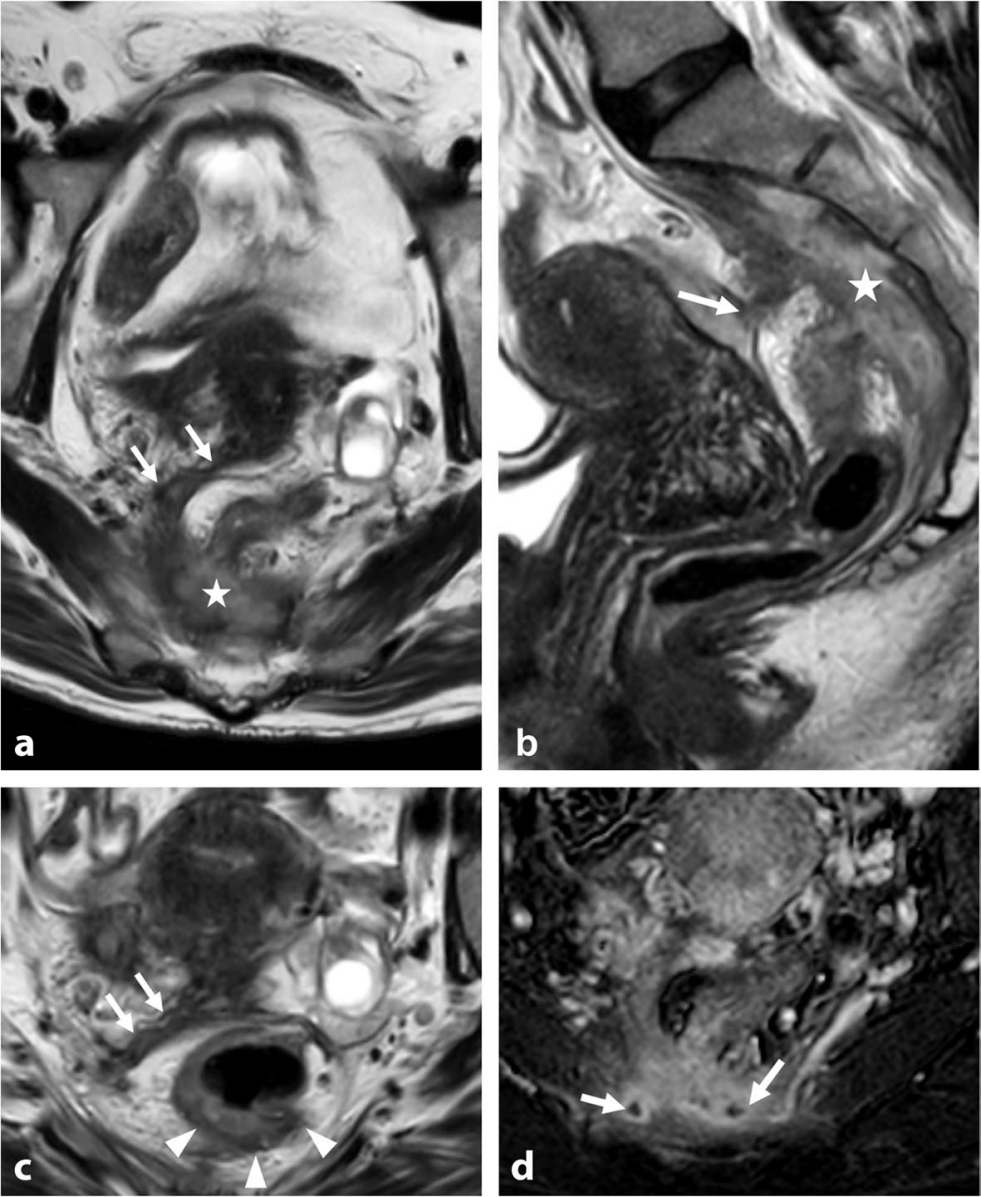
Actinomycosis in a 36-year-old woman with chronic pelvic pain, rectal disorder. and dyspareunia. a Axial and (b) sagittal T2-W MR images show right subperitoneal infiltration with intermediate signal intensity, centered on the right utero-sacral ligament (thin arrows) and the sacro-recto-genital septum up to the presacral space (star) from the first sacral vertebra to the sacrococcygeal junction. c Axial T2-W MR image shows perirectal soft-tissue infiltration (arrows) with intermediate T2-signal intensity semicircumferential thickening of the upper and posterior rectum (arrowheads). d Axial T1-W fat-suppressed contrast-enhanced subtracted MR image shows avid enhancement of the surrounding inflammatory infiltration with small abscesses in the presacral space (arrows). Past medical history of intrauterine device recent removal was found
